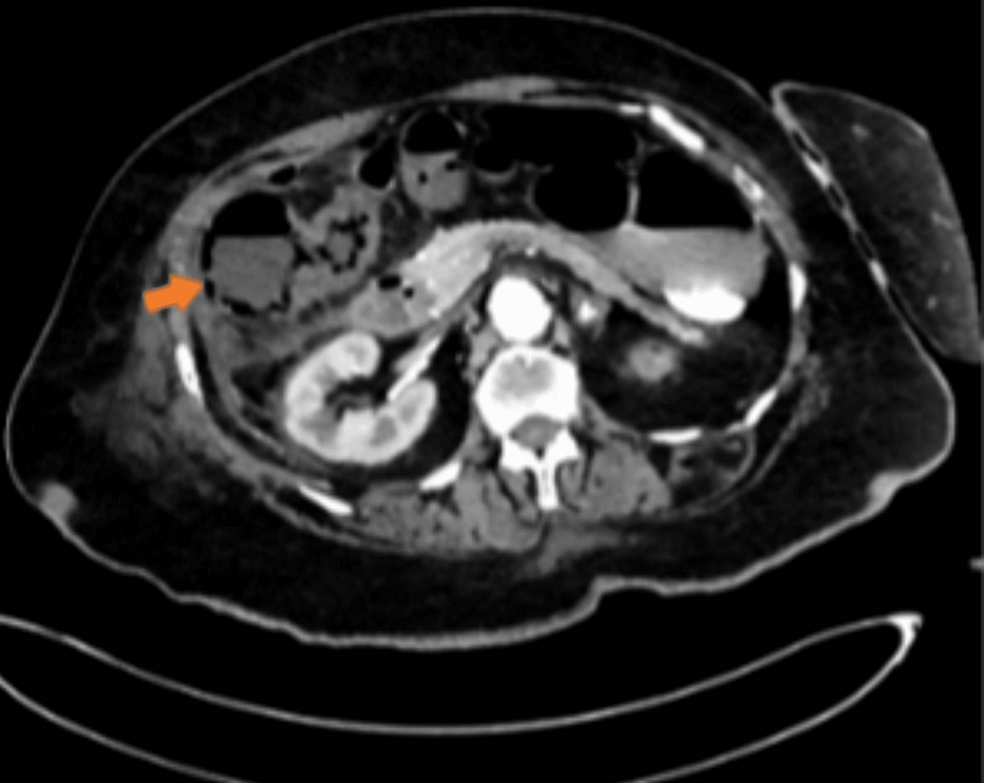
Axial CT angiogram of the abdomen and pelvis showing Pneumatosis intestinalis (orange arrow)
